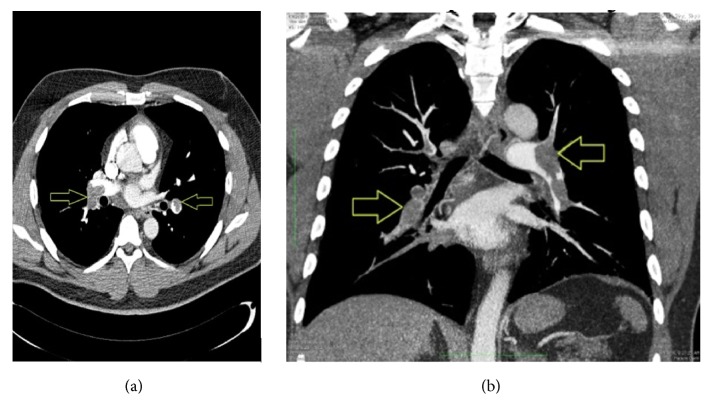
(a) CT pulmonary angiogram axial image showing a large filling defect in the right and left main pulmonary arteries affecting supply to both lobes of the lungs (see arrows). (b) CT pulmonary angiogram coronal view depicting large filling defects in the right and left main pulmonary arteries and bilateral lobar pulmonary arteries with bilateral lower lobe pulmonary atelectasis (see arrows).
